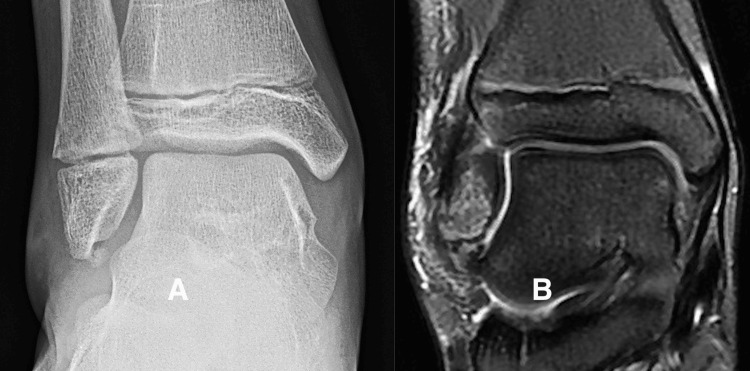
Low-intensity signal between the subfibular ossicle (SO) and tip of the fibula Figure 2A is a radiograph showing an SO at the tip of the fibula. Figure 2B is a coronal T2-weighted MRI sequence showing a low-intensity signal in the gap between the SO and the tip of the fibula. The child had recently sustained an inversion ankle sprain, and the MRI sequences showed soft tissue and bone edema related to the recent injury but no increased signal intensity between the SO and the fibula.
